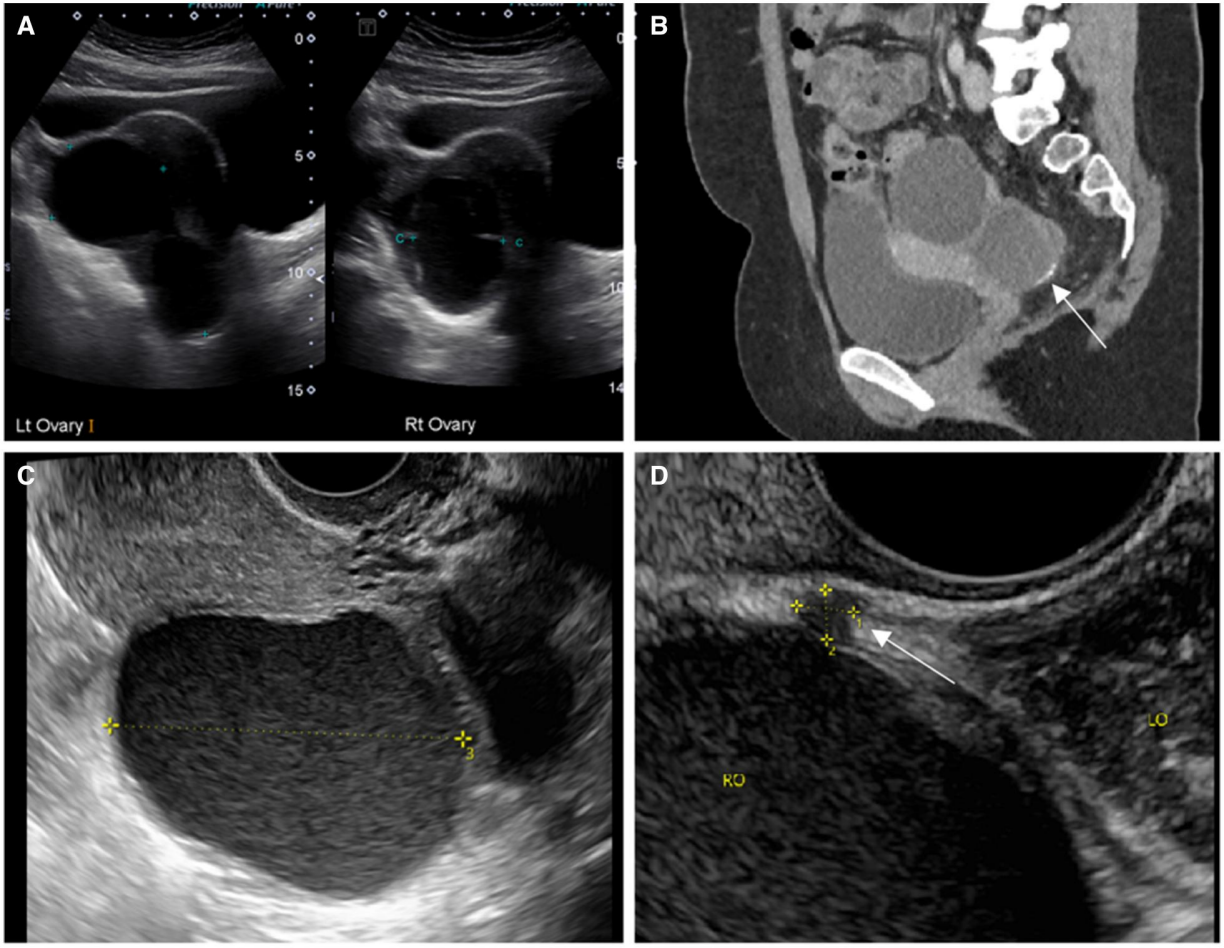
A 29-year-old female presented to the emergency department with sudden onset of right lower abdominal pain and nausea. (A) Transabdominal US showed enlarged ovaries with multiple cysts. (B) Computed tomography (CT) showed multiple cysts arising from both ovaries with rim calcification demonstrated in one of the left ovarian cysts (arrow). TVUS performed 14 days later showed multiple endometriomas, measuring up to 49mm, with internal echoes and fluid-fluid levels(C). A DE assessment (D) further demonstrated a 4mm right uterosacral ligament nodule (arrow).
